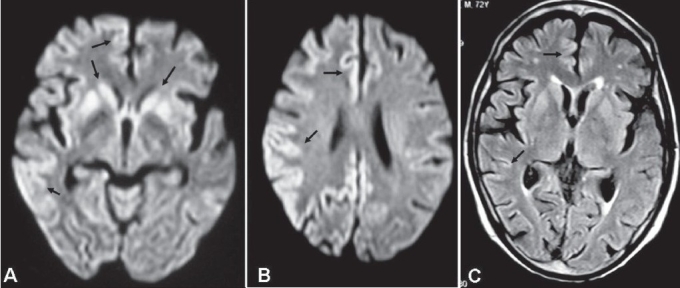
Diffusion weighted MRI sequence (A and B) shows symmetrical hyperintensity involving bilateral caudate nucleus, globus pallidus, putamen, and cerebral cortex (arrows). The FLAIR image (C) also shows diffuse hyperintensity involving the cerebral cortices and basal ganglia (arrows)
